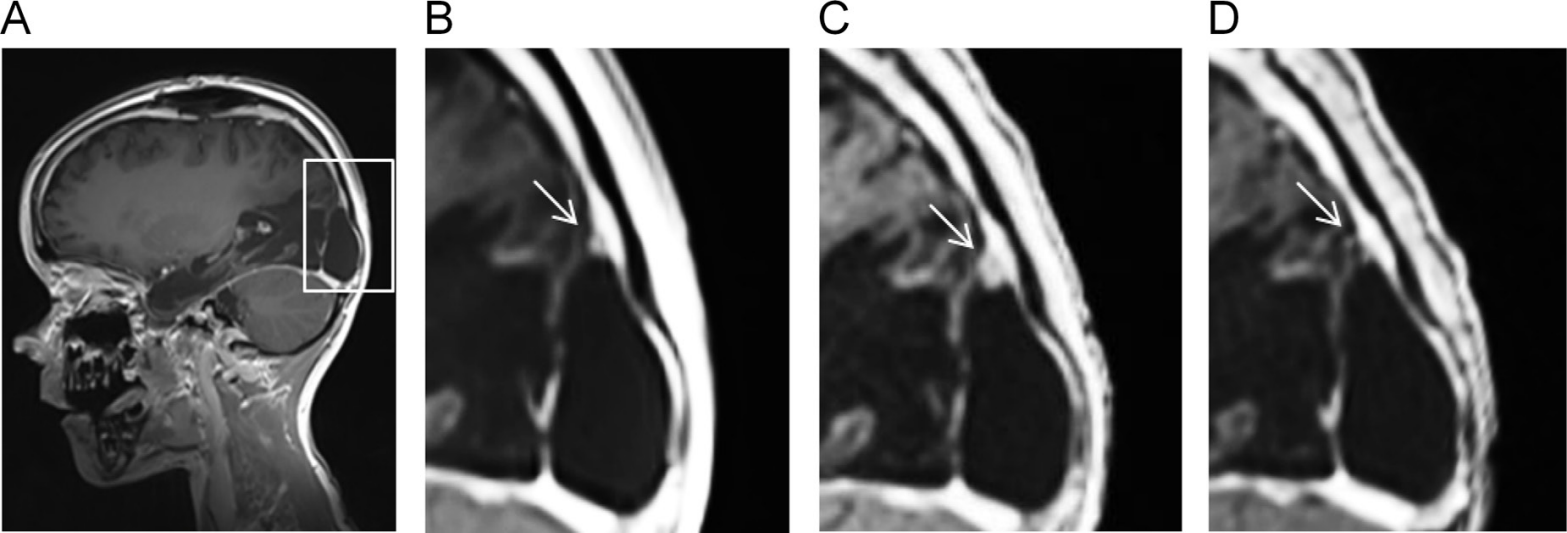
The target lesion is sensitive to the targeted therapy MRI showing the dural lesion developed under the first line therapy (A-B), before the start of the targeted therapy (C) and at the time of CR (D).
